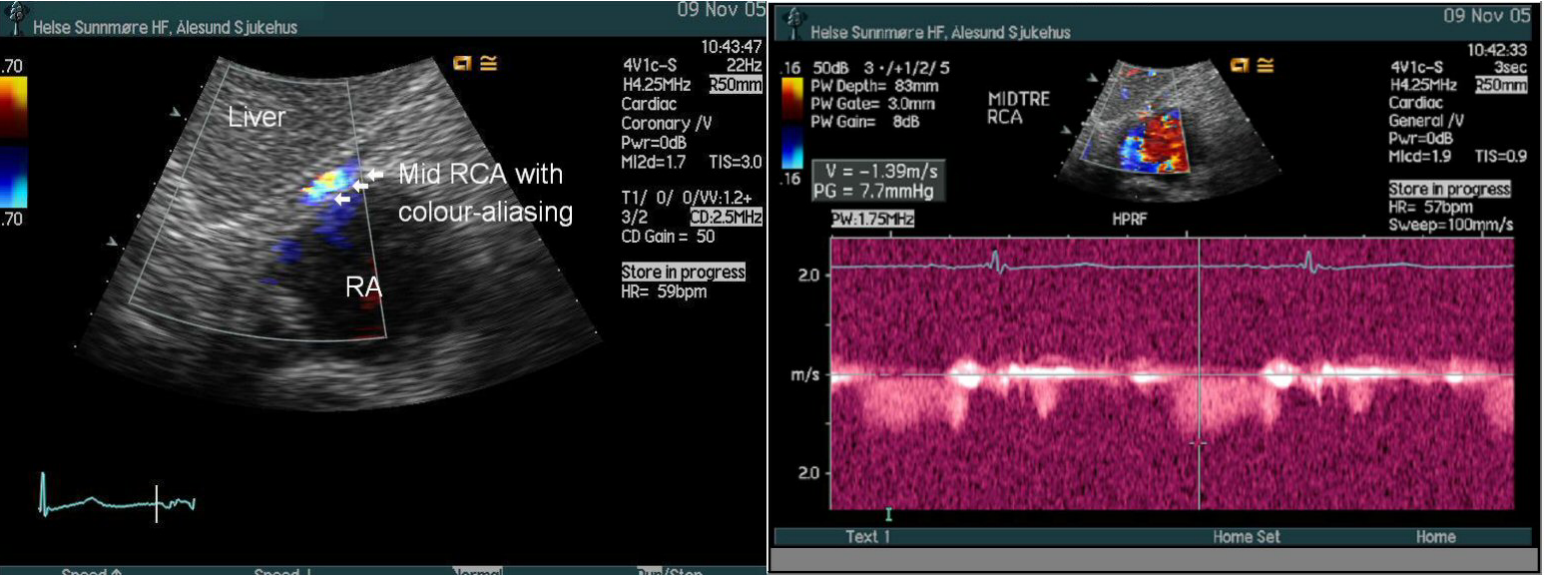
In the modified subcostal four-chamber window we identified a segment of the mid RCA with colour-aliasing suggestive for a stenosis. Stenotic diastolic peak velocity measured with pulsed wave Doppler 1.39 m/s, in a modified subcostal four chamber view.
